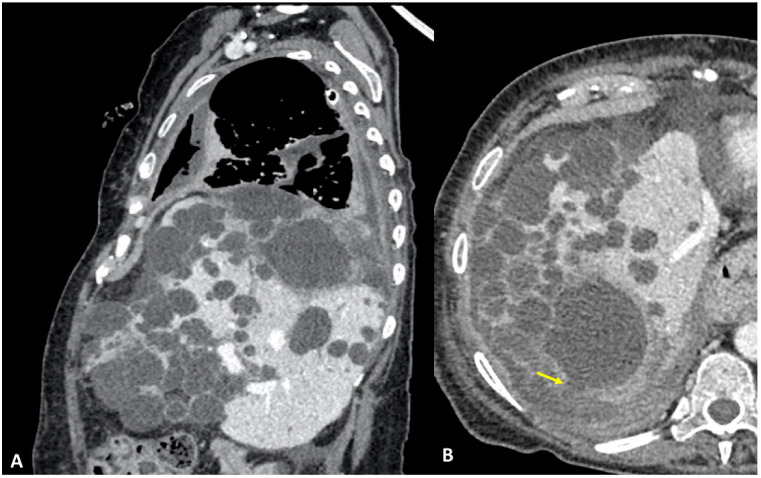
(A,B) Contrast-enhanced CT, sagittal planes (A), and axial planes (B) showing right empyema and pleural thickening with enhanced pleural leaflets. In both figures (A,B), the right lower lung lobe and a hepatic cyst look strongly adjacent (arrow), without a definite fistulous tract.
